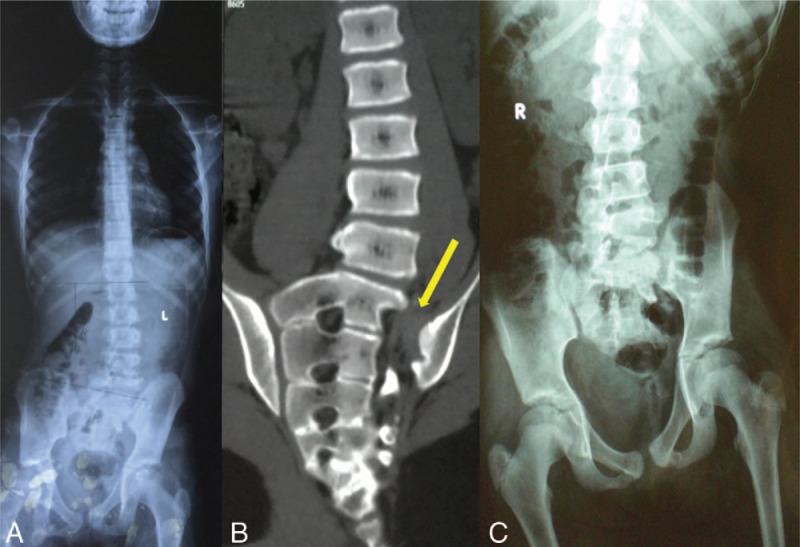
(A) X-ray of spine and pelvis in the supine position. (B) CT reconstruction revealed left sacral agenesis and displacement. (C) In the standing position, the patient's left sacrum was higher than the right with obvious pelvis tilt. CT = computed tomography.
